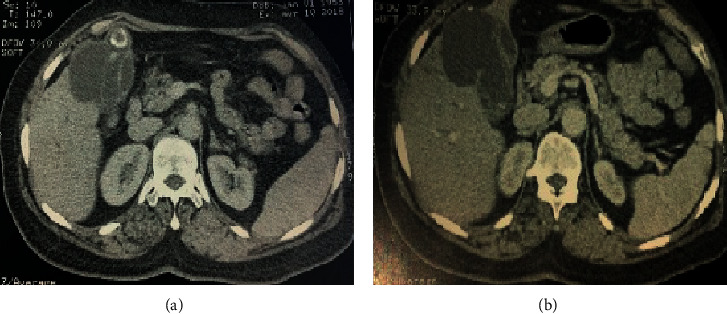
(a, b) Computed tomography (CT) scan showing a fistula between hydatid cyst and gallbladder.
